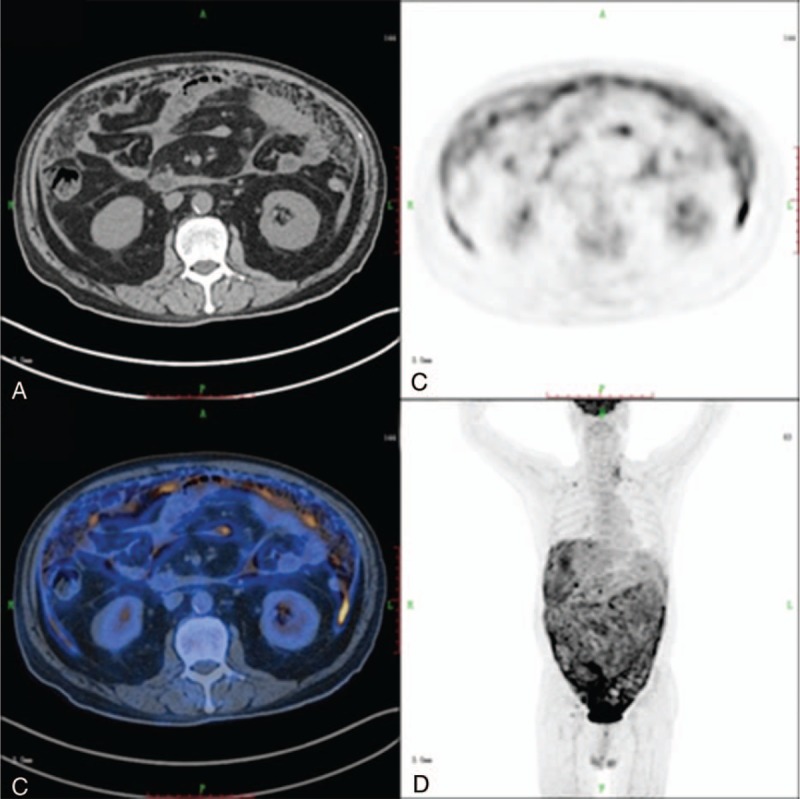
Images of 73-year-old man who went in for check-up: axial CT (A), axial PET (B), axial fused PET/CT (C), and 3D PET (D). The patient had serum CA12-5 of 246 U/mL, normal carcinoembryonic antigen, and normal CA19-9. PET/CT images show high uptake in the peritoneum (SUV_max_ of 4.6) which suggest a malignant lesion. After PET/CT examination, peritoneal biopsy confirmed that the patient had malignant peritoneal mesothelioma. CT = computed tomography, PET = positron emission tomography, SUV_max_ = maximal standardized uptake value.
